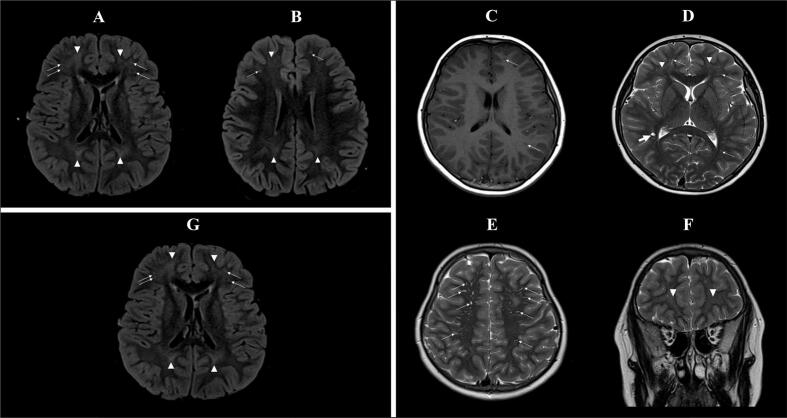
Magnetic Resonance Imaging (MRI) performed at admission (A, B) at day 5 (C, D, E, F) and 6 months after vitamin supplementation (G). A. Axial T2 FLAIR weighted imaging during the acute phase (at admission): symmetric mild increased FLAIR signals in bilateral fronto-parietal white matter (arrowheads), associated with punctiform high FLAIR signals in the frontal white matter, corresponding to perivascular spaces (arrows). B. Axial T2 FLAIR weighted imaging during the acute phase (at admission): symmetric mild increased FLAIR signal in bilateral fronto-parietal white matter (arrowhead), associated with punctiform high FLAIR signals in the frontal white matter (arrows), corresponding to perivascular spaces. C. Axial T1 weighted imaging 5 days after admission: no associated decreased T1 signal in bilateral fronto-parietal white matter lesions; visibility of some linear perivascular spaces (arrows). D. Axial T2 weighted imaging 5 days after admission: symmetric mild increased T2 signal in bilateral fronto-parietal white matter (arrowheads), associated with numerous linear (arrows) or cystic (bold arrow) perivascular spaces. E. Axial T2 weighted imaging 5 days after admission: numerous linear perivascular spaces (arrows). F. Coronal T2 weighted imaging 5 days after admission: symmetric mild increased T2 signal in bilateral anterior frontal white matter (arrowheads). G. Axial T2 FLAIR weighted 6 months after vitamin supplementation: stability of the symmetric mild increased FLAIR signal in bilateral fronto-parietal white matter (arrows), associated with punctiform high FLAIR signals in the frontal white matter (arrowheads), corresponding to known enlarged perivascular spaces.
